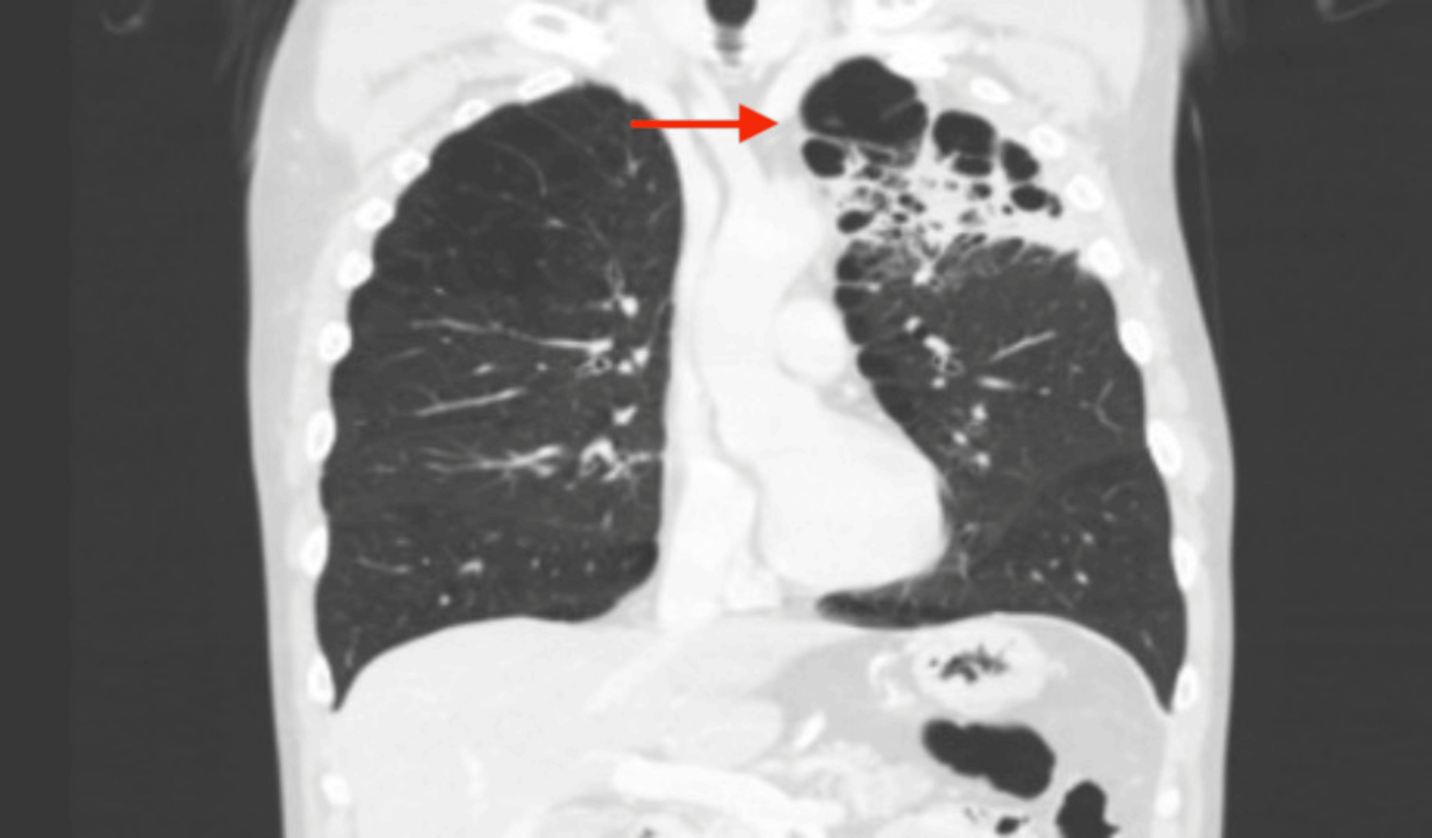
Computed tomography (CT) scan of the thorax An arrow highlights an early-stage cavity, initially presenting as inflammatory changes in the left upper lobe, with fluid accumulation visible within an emphysematous bulla.
